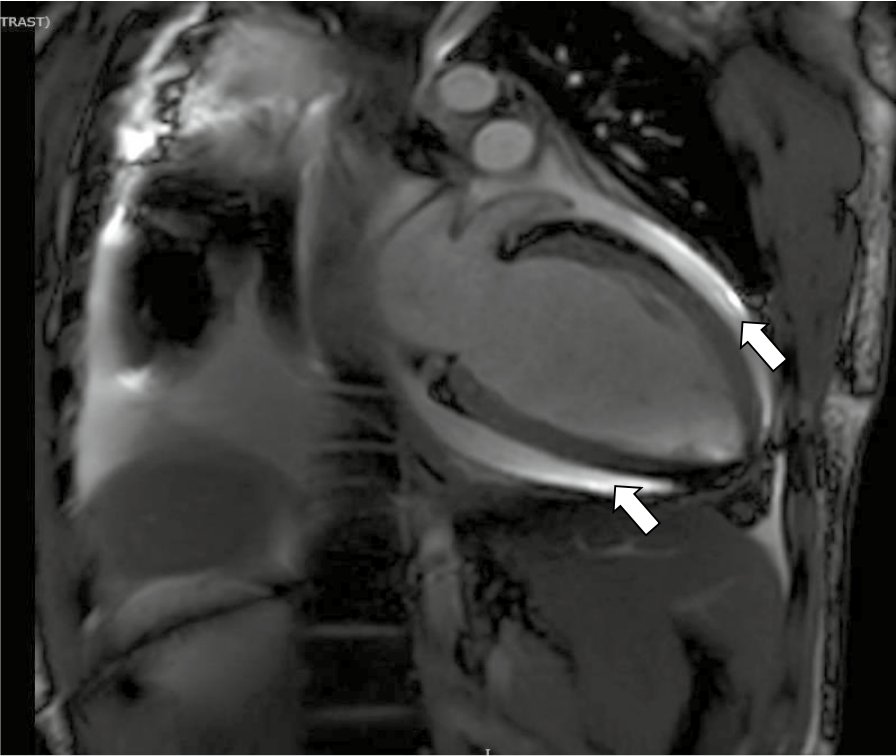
A Cardiac MRI, T-2 weighted axial image of diastolic phase with late gadolinium enhancement sequence, demonstrating left ventricular dilation and large bilateral pleural effusions and pericardial effusions (white arrows). Myocardial tissue characterization with late gadolinium hyperenhancement was unable to be performed due to patient’s inability to lie recumbent and tachypnea
